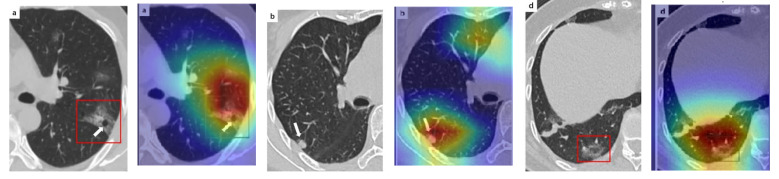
Example of annotated CT scans with different manifestations of COVID-19 taken from [61], and their corresponding localization maps. Our models were able to identify them as COVID-19 cases and accurately localize their COVID-19-associated regions.
